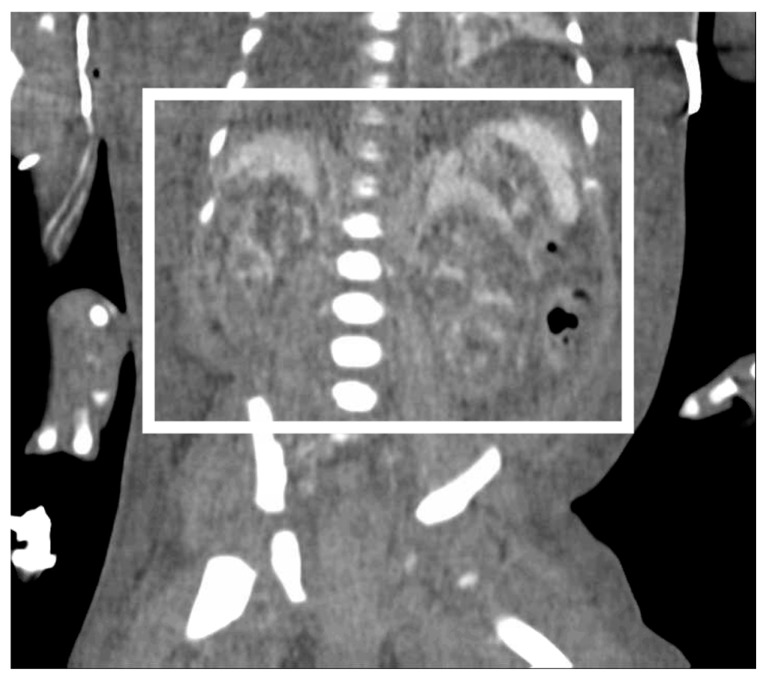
Computed tomography image utilizing intravenous contrast on day of life 5 showing bilateral patchy opacification of the external renal capsule and central renal medulla (box).
